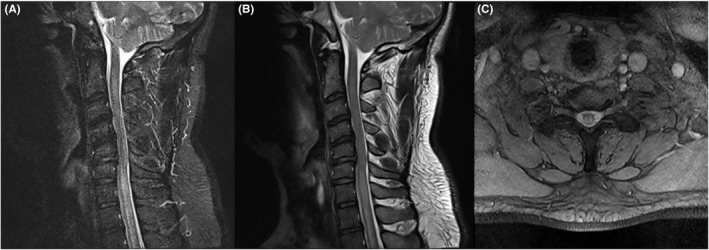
Cervical magnetic resonance imaging of first patient: longitudinally extensive transverse myelitis involving second cervical segment to second thoracic segment of spinal cord. (A) Sagittal section, Short‐TI Inversion Recovery sequence. (B) Sagittal section, T2 sequence. (C) Axial section, T2 sequence: hyperintensity involving more than 2/3 of axial section
